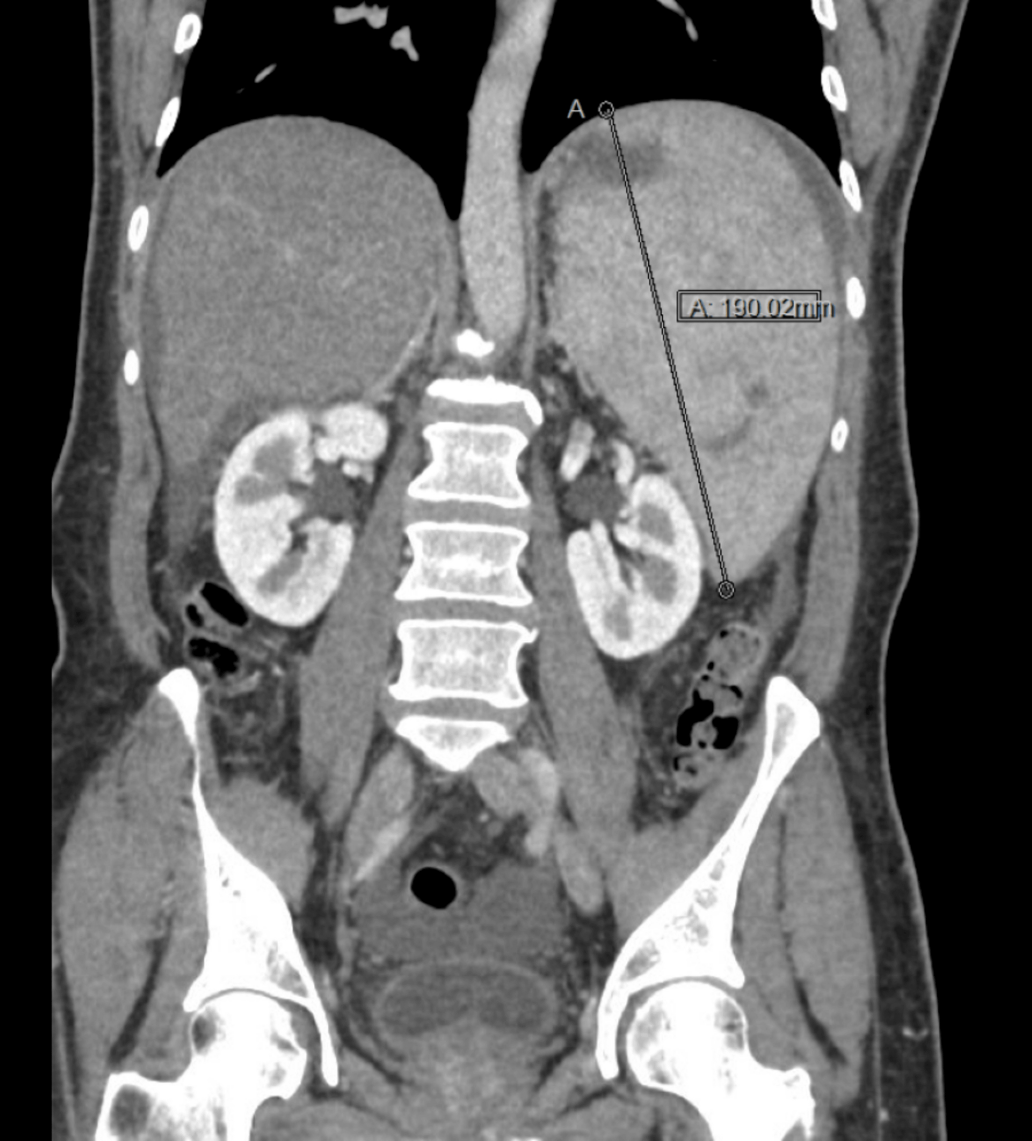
CT abdomen revealed an enlarged spleen of about 190 mm (measurement indicated by the black line)
